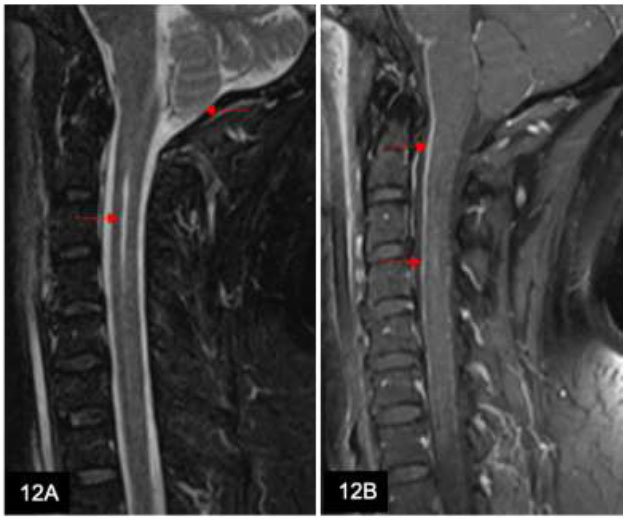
A 61-year-old man presents with acute urinary retention, fever, and hyperreflexia in all limbs. Sagittal T2w MRI of the cervical spine (A) shows cerebellar tonsillar descent to 5 mm beyond the foramen magnum and a long segment high T2 signal within the central spinal cord (arrows). These findings suggest a Chiari type I malformation with syringomyelia in the cervical cord. Sagittal T1w post-contrast MRI (B) shows congestion of the epidural venous plexus, mimicking leptomeningeal enhancement (arrows).
